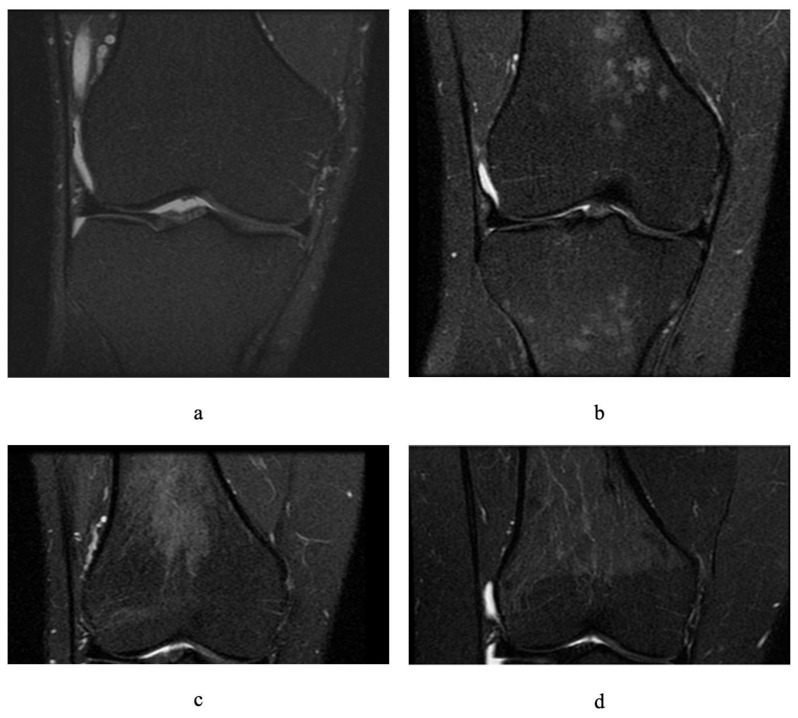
Different grades of residual or reconverted red bone marrow on fat-saturated proton-density-weighted magnetic resonance images of adult knees (coronal sections): (a) grade 0 in femur and tibia, (b) grade 1 in femur and tibia, (c) grade 2 in femur, (d) grade 3 in femur.
